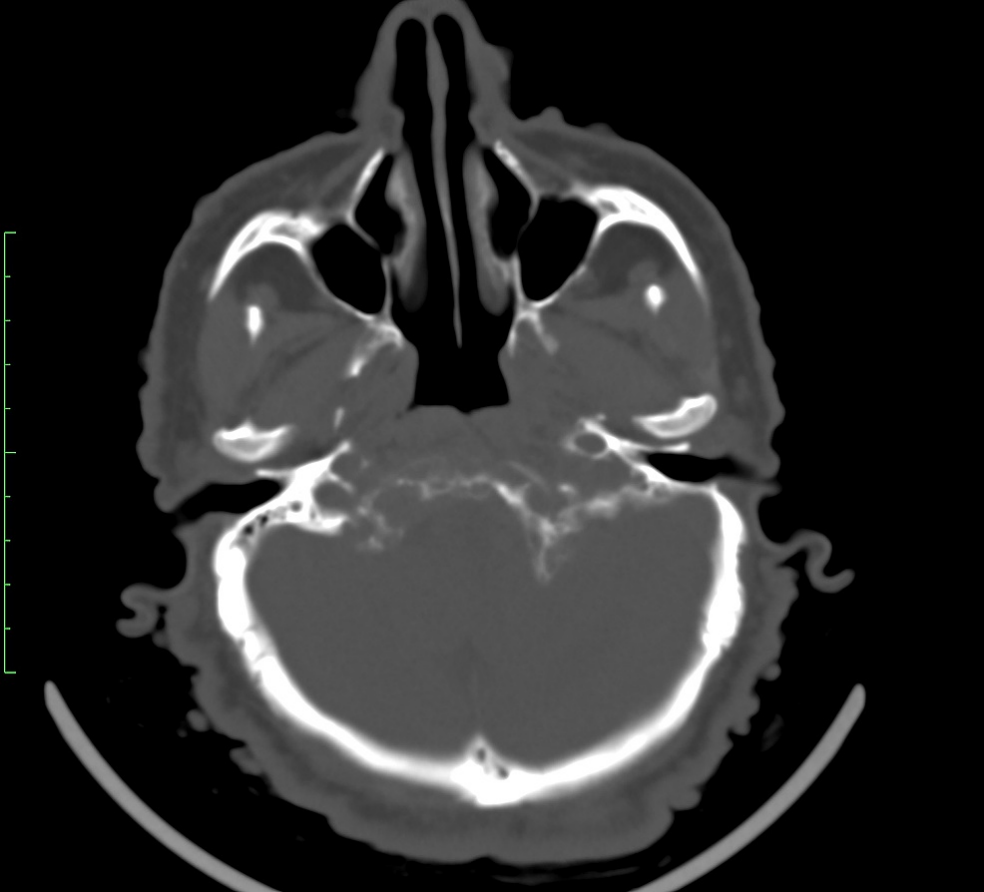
Computed tomography scan showing the aggressive destruction of the bones of the base of the skull.
